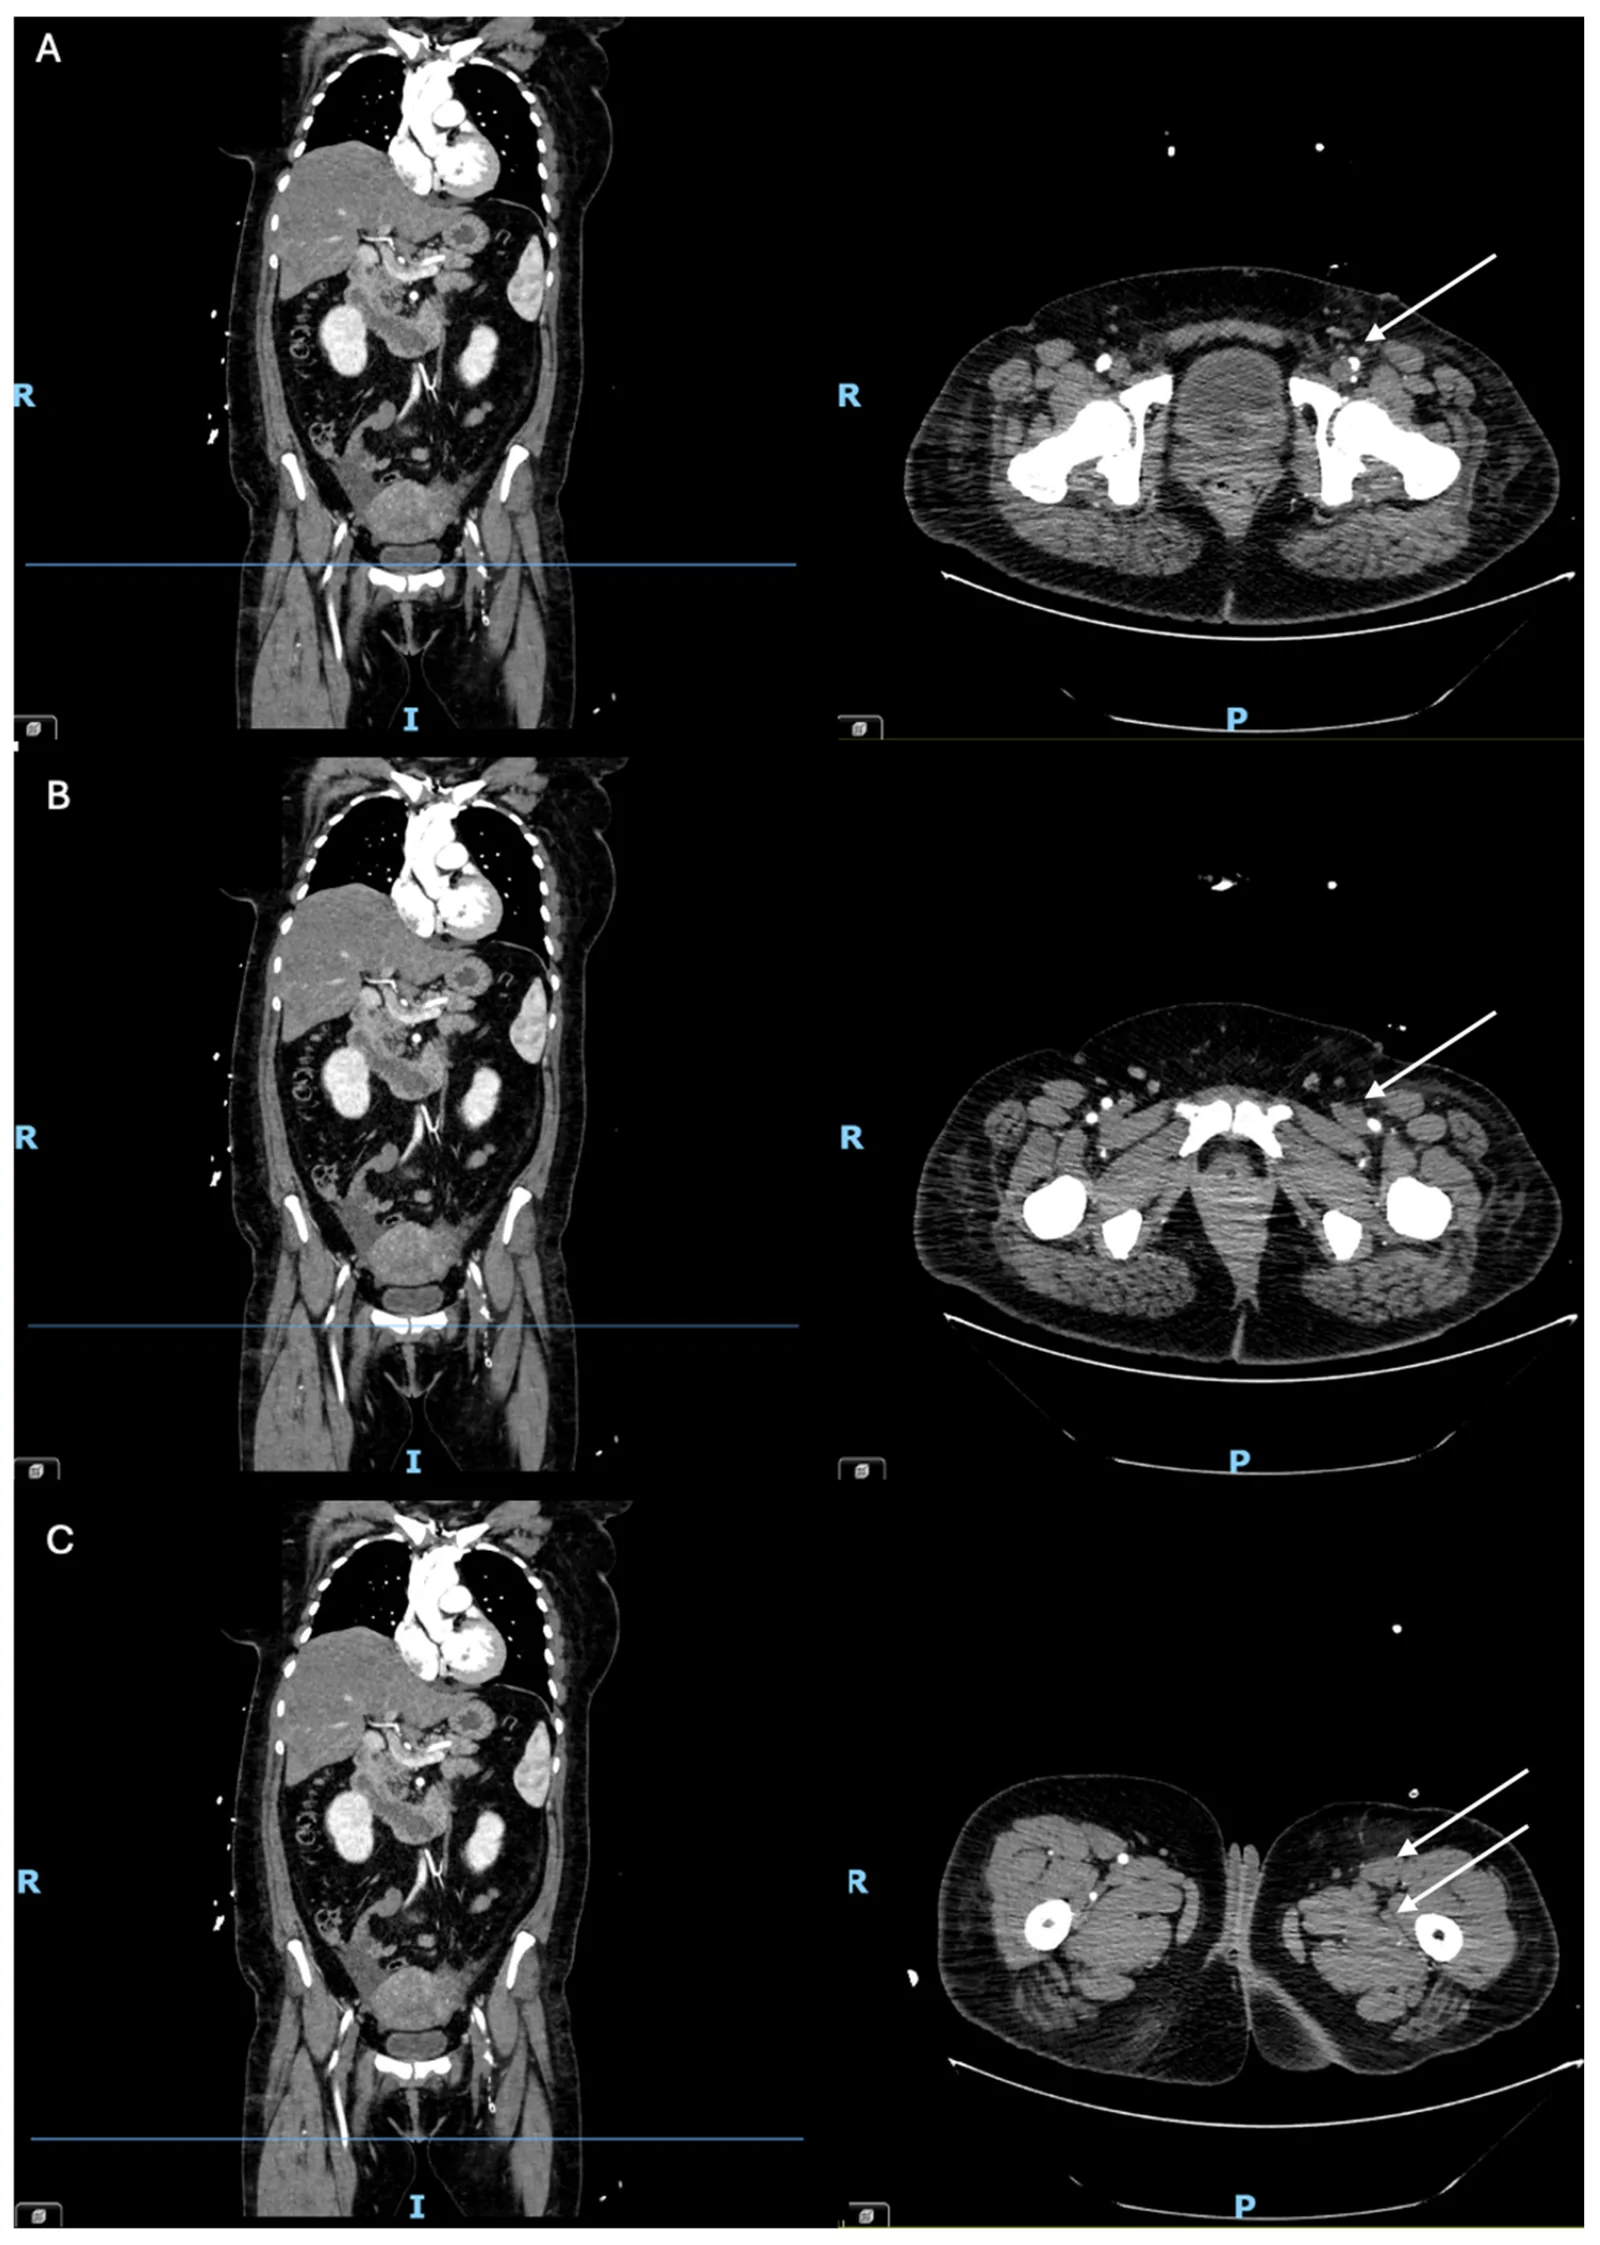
Computed Tomography Angiography performed in the reported case. The arrows indicate intraluminal filling defects in the arteries of the left lower limb on arterial-phase contrast-enhanced Computed Tomography Angiography. In panels (A–C), the axial image is shown on the right and the corresponding coronal reconstruction on the left; the horizontal blue line in the coronal images indicates the level of the corresponding axial sections. Patient orientation is indicated by R (right), I (inferior), and P (posterior). (A) Partial thrombotic occlusion of the left common femoral artery. (B) Patency of the proximal left deep femoral artery, with occlusion of the left superficial femoral artery. (C) Occlusion of the mid segment of the left deep femoral artery, with persistent occlusion of the left superficial femoral artery.
